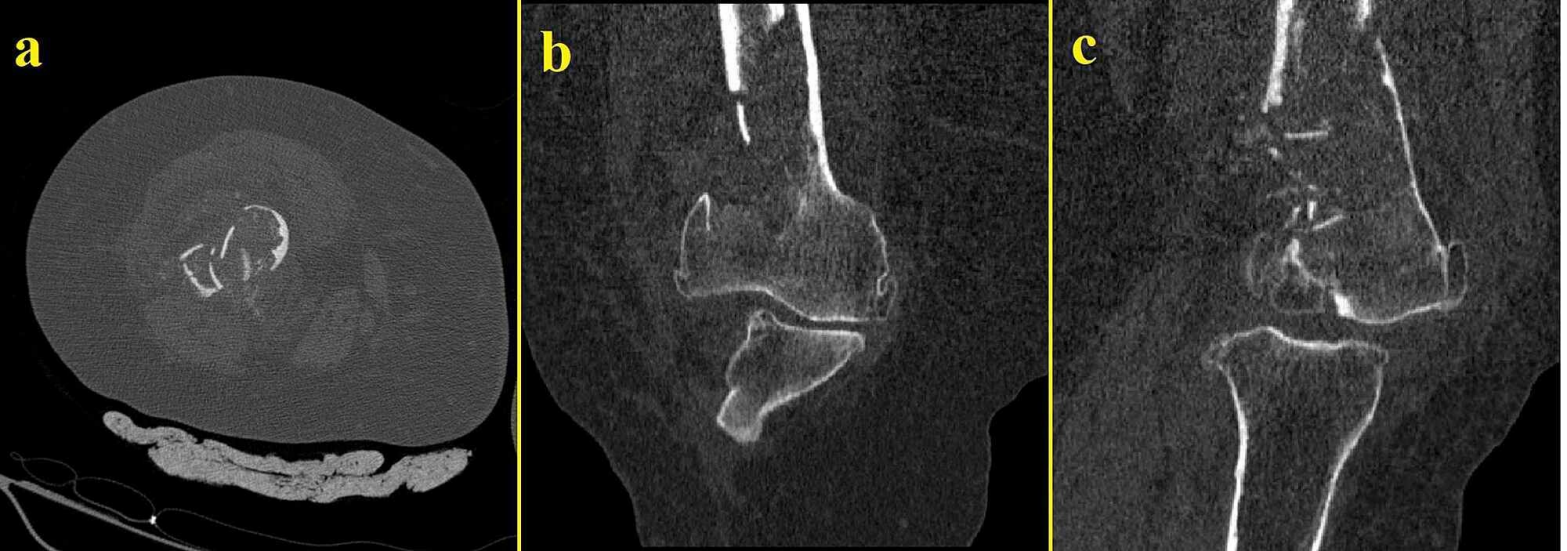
Initial CT scans of the same extremity taken on the day of trauma Thinning of the cortexes and lysis can be observed CT: computed tomography
